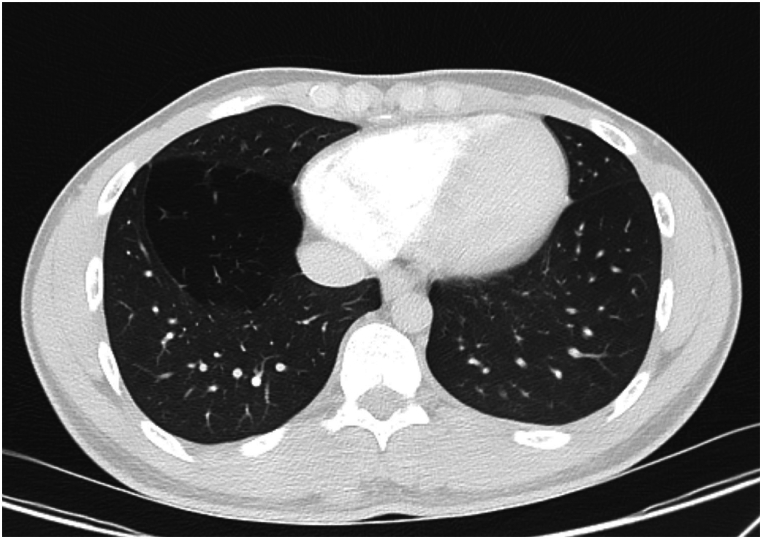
Axial CT image. Hyperinflated medial basal segment of right lower lobe.
